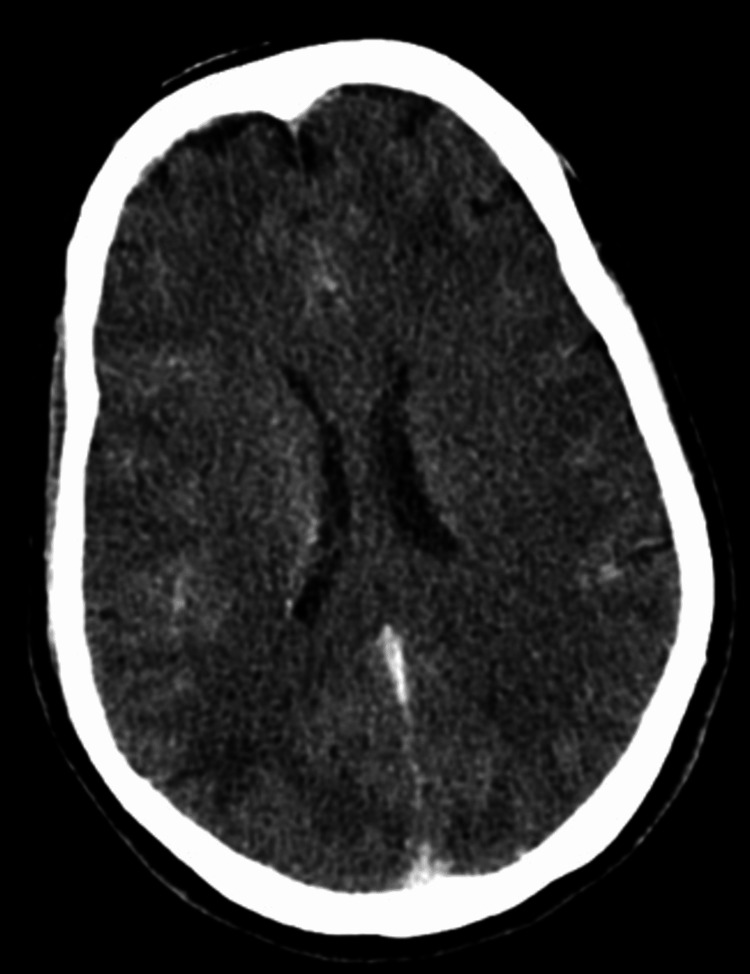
Contrast-enhanced CT of the brain showing no focal lesions or cortical venous thrombosis.
